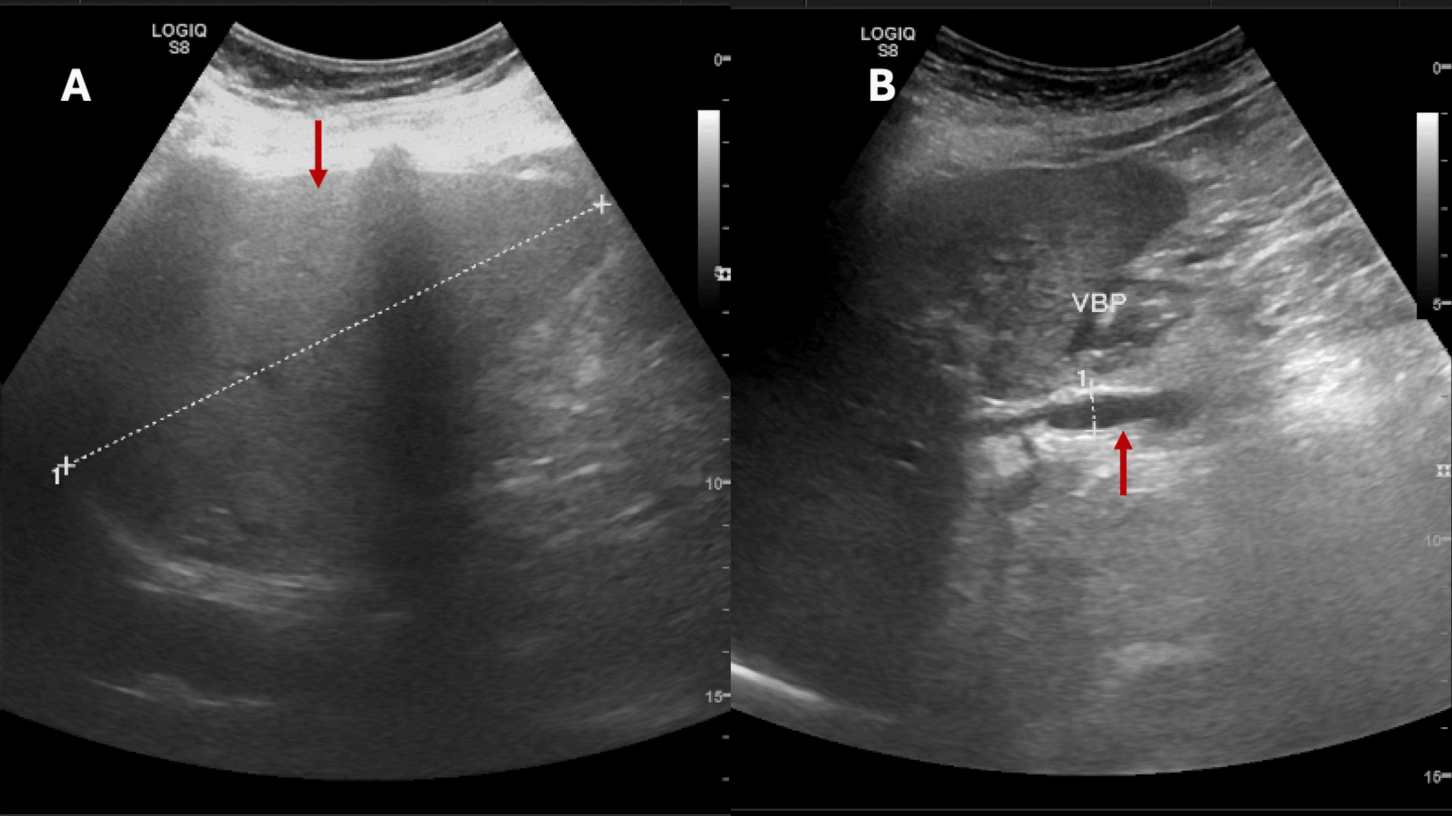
Abdominal ultrasound images obtained during the diagnostic workup. (A) Longitudinal view of the liver showing a slightly heterogeneous but globally preserved echotexture, with no focal lesions or perihepatic fluid (arrow). Findings were interpreted as suggestive of diffuse steatosis. (B) View of the extrahepatic biliary tract showing a mildly dilated common bile duct, measuring approximately 9 mm (arrow), without intrahepatic biliary dilation.
